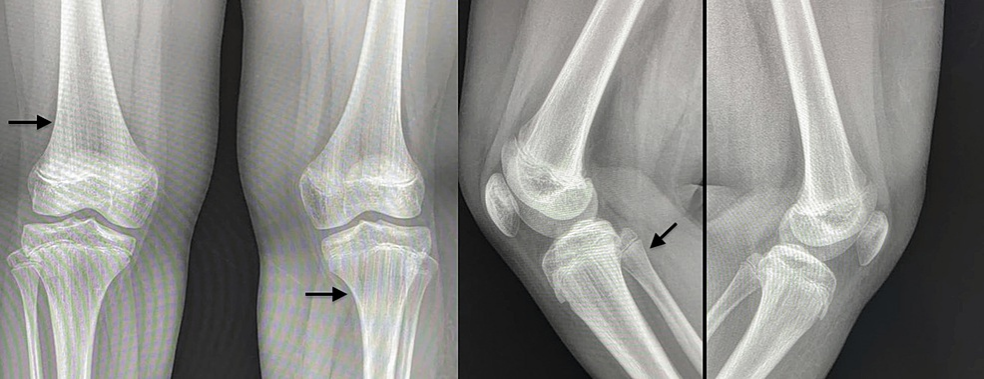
Plain X-ray showed osteopathic striata with prominent vertical striations in metaphysis into diaphysis of femur, tibia and fibula bilaterally
